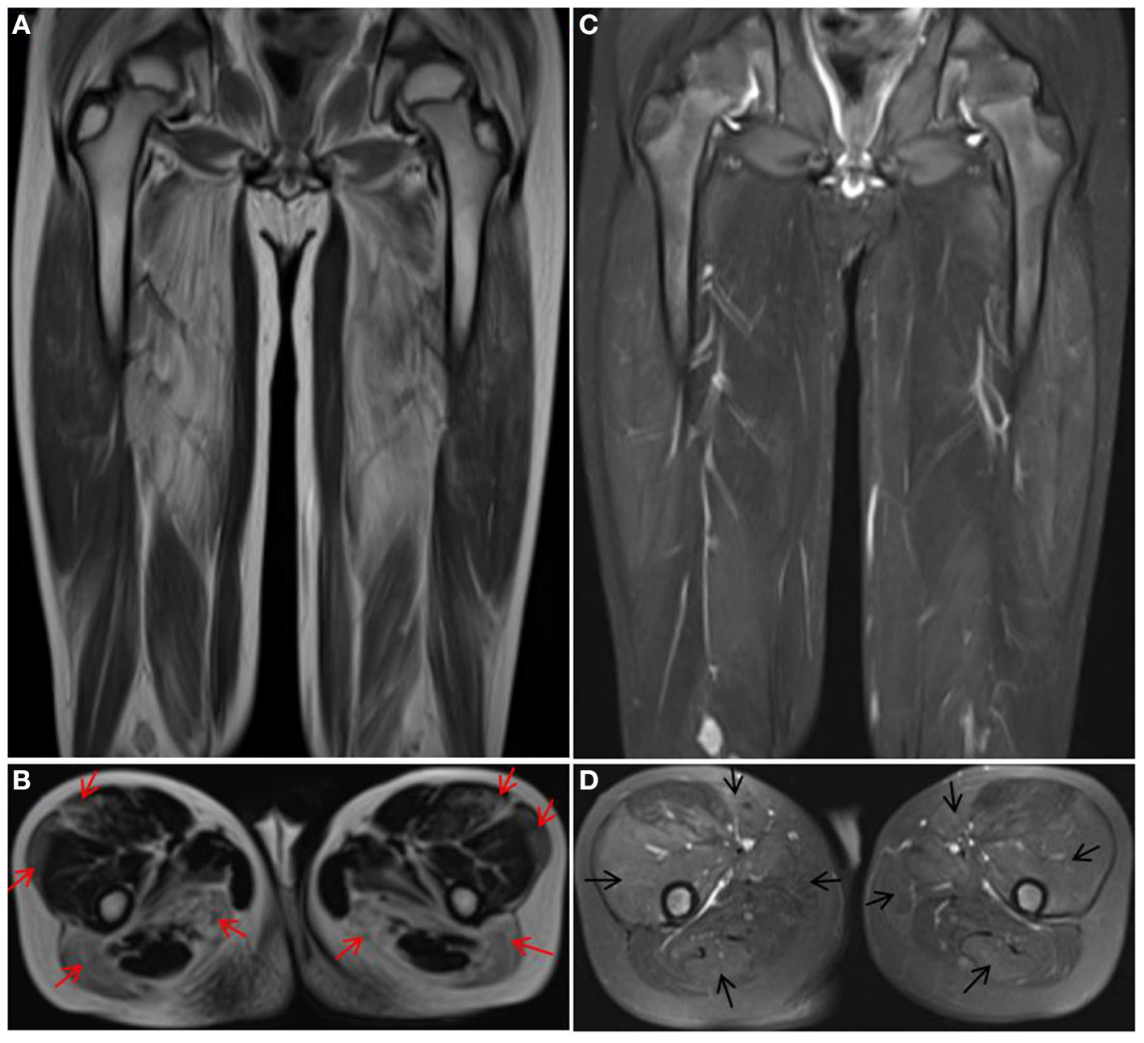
Muscle MRI of case 1. T1 (A,B) showed obvious fat infiltration in thigh muscles, most notably in the vastus lateralis, rectus femoris, biceps femoris, and semimembranosus muscles (red arrow), but the fat infiltration in the vastus intermedius, sartorius, gracilis, and semitendinosus muscles was mild. T2 (C,D) showed obvious inflammation edema in the muscles with mild fat infiltration (black arrow).
